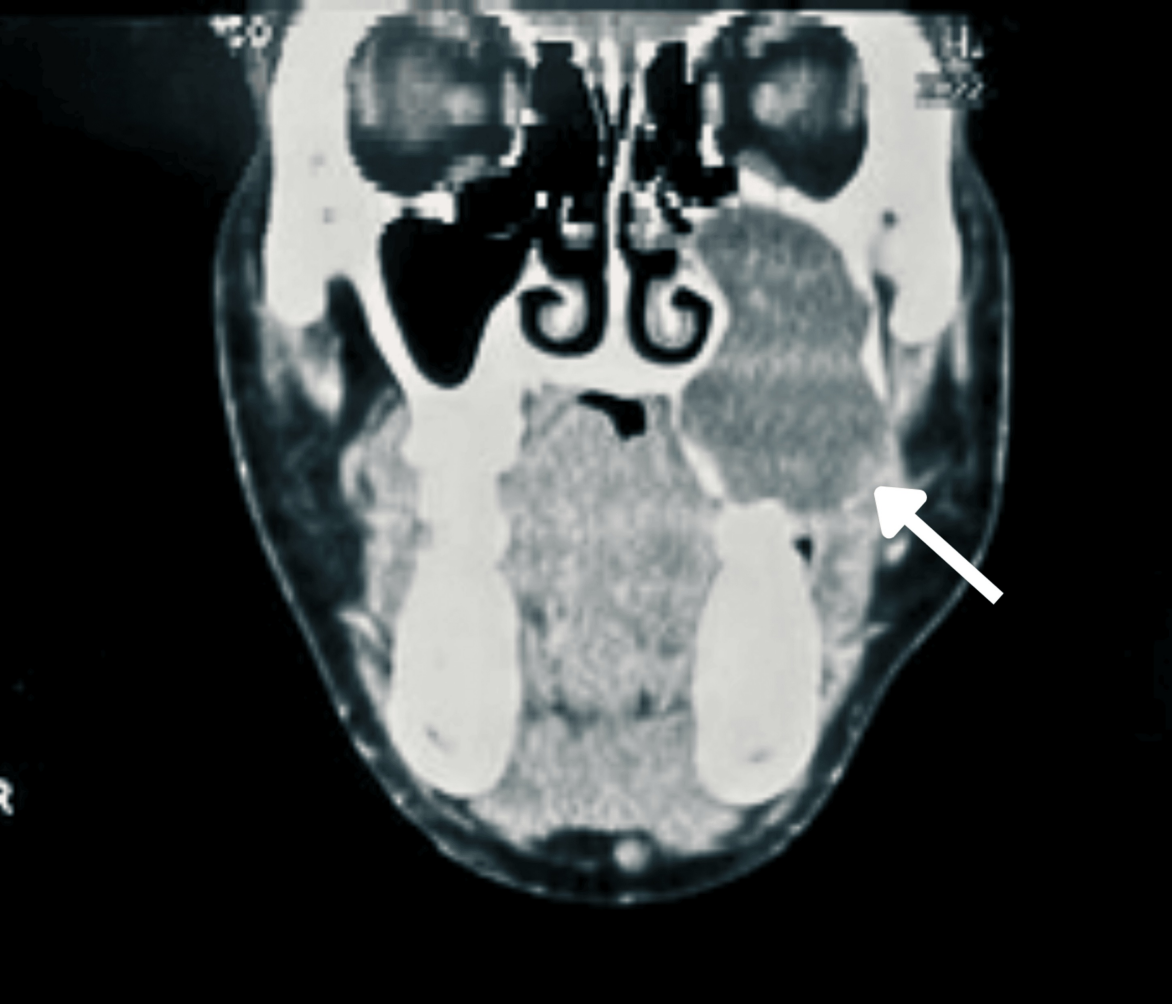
The CT scan (coronal view) shows a lesion occupying the left maxillary sinus and cortical defects over the inferior aspect of the left maxillary sinus (white arrow).
